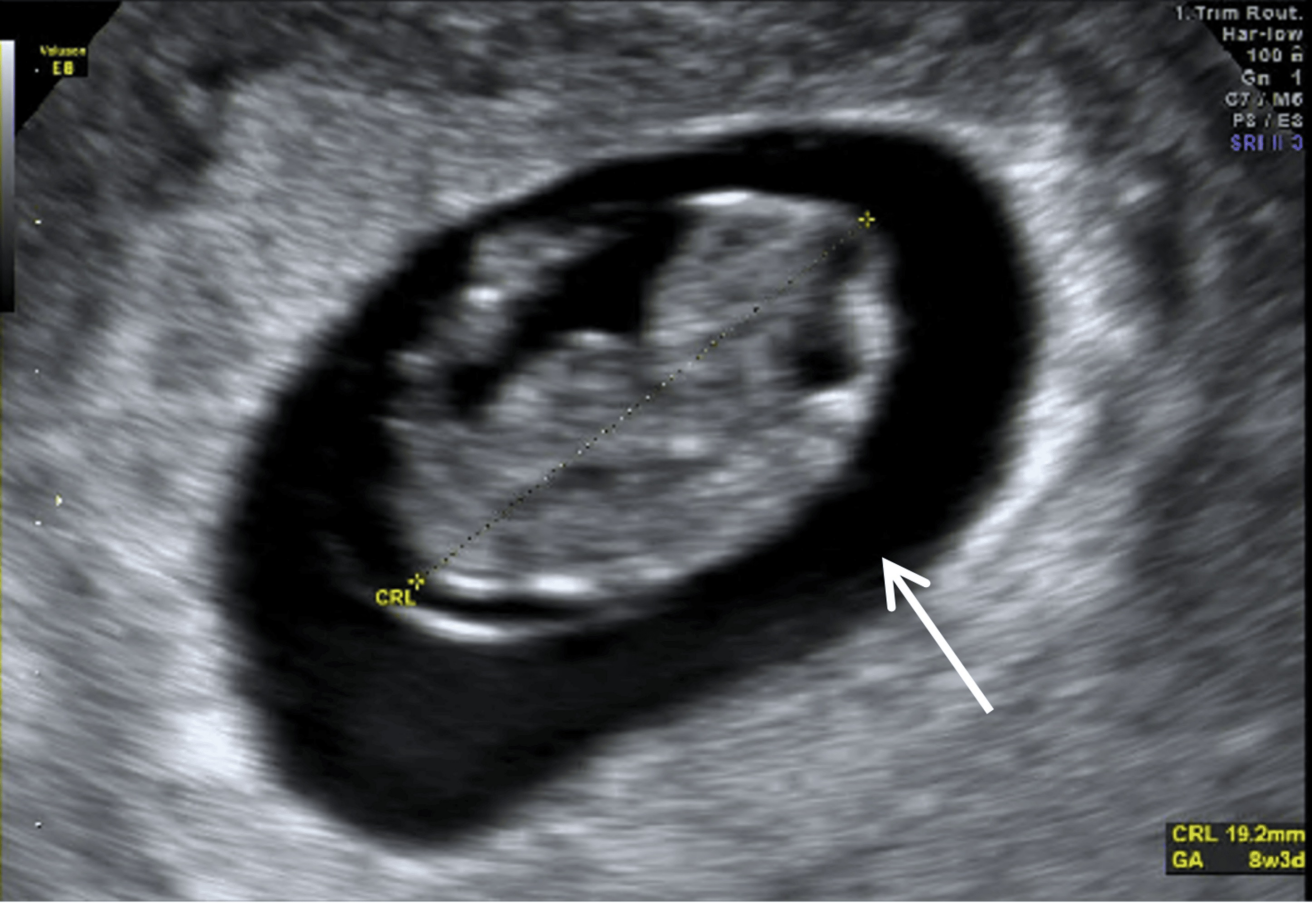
Latest transvaginal ultrasound performed at our clinic, showing adequate fetal growth The white arrow indicates an intrauterine gestational sac with a CRL corresponding to eight weeks and three days. CRL, crown-rump length; GA, gestational age
